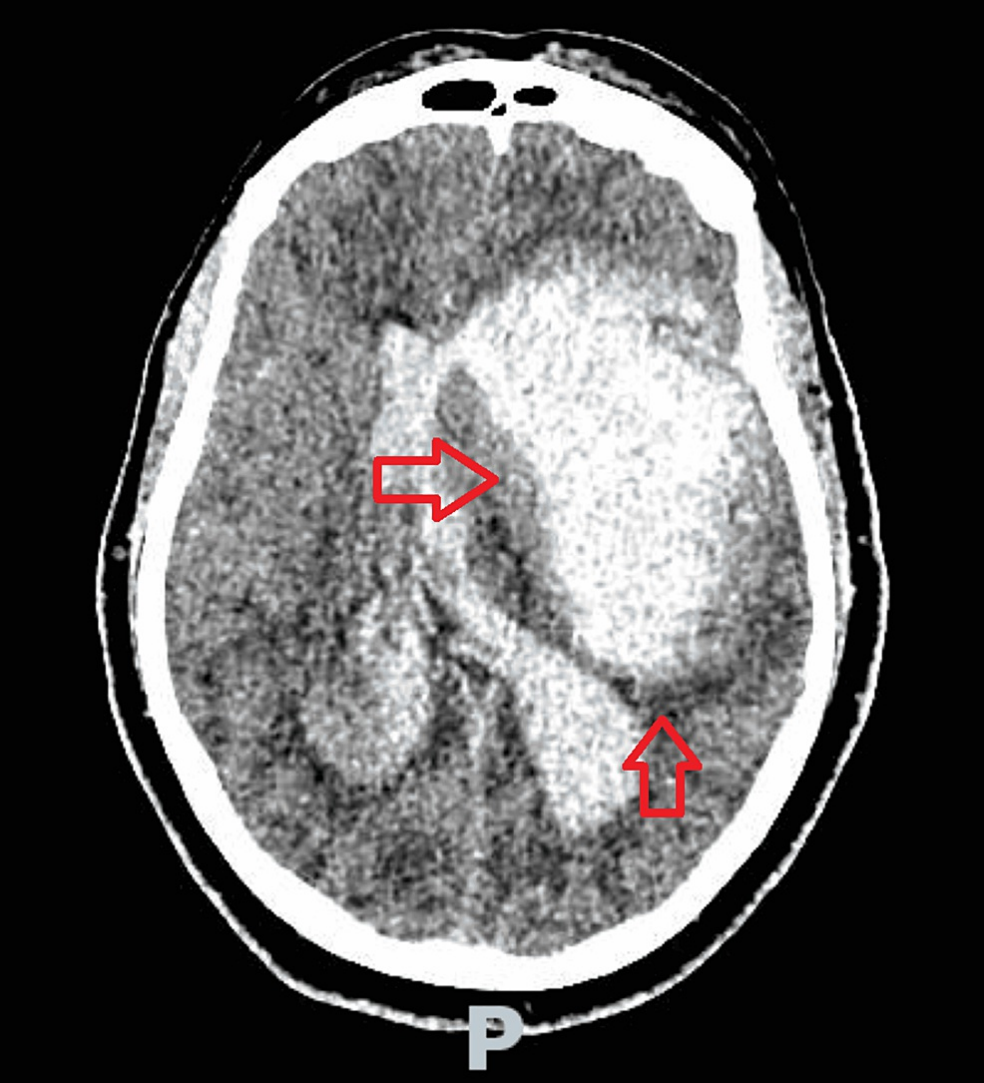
Computed tomography of the head showing large intraparenchymal hemorrhage of the left anterior temporal lobe and left inferior frontal lobe (arrows) with surrounding edema and mass effect.
